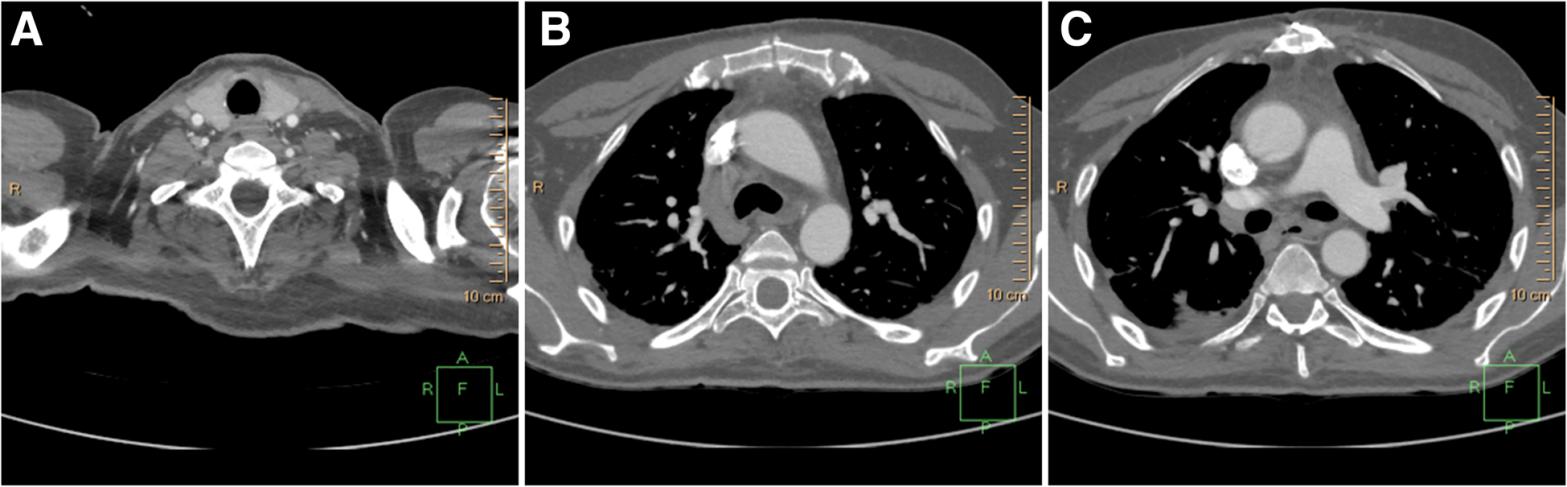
Control computed tomographic analysis. a Control computed tomographic scan obtained on day 44 shows normal physiological findings indicating a complete regression of the abscess formation near the left lobe of the thyroid gland and ventral of the carotid arteries. b and c Fibrous adhesion in the middle mediastinal space
